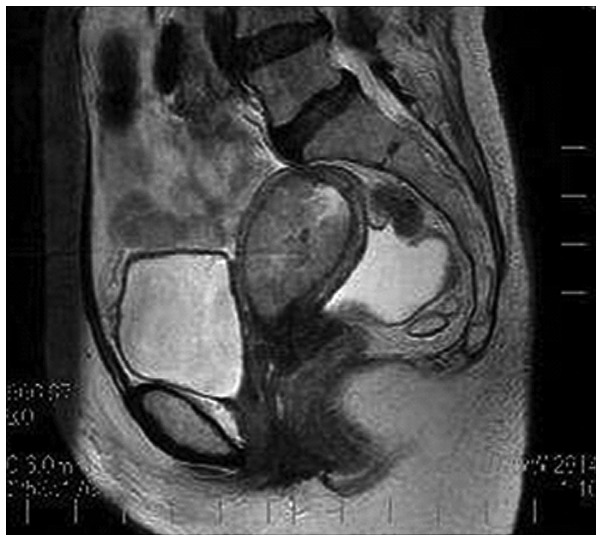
Magnetic resonance imaging showing thickening of the endometrium and a tumorous lesion with enhancement in the uterine corpus wall.
